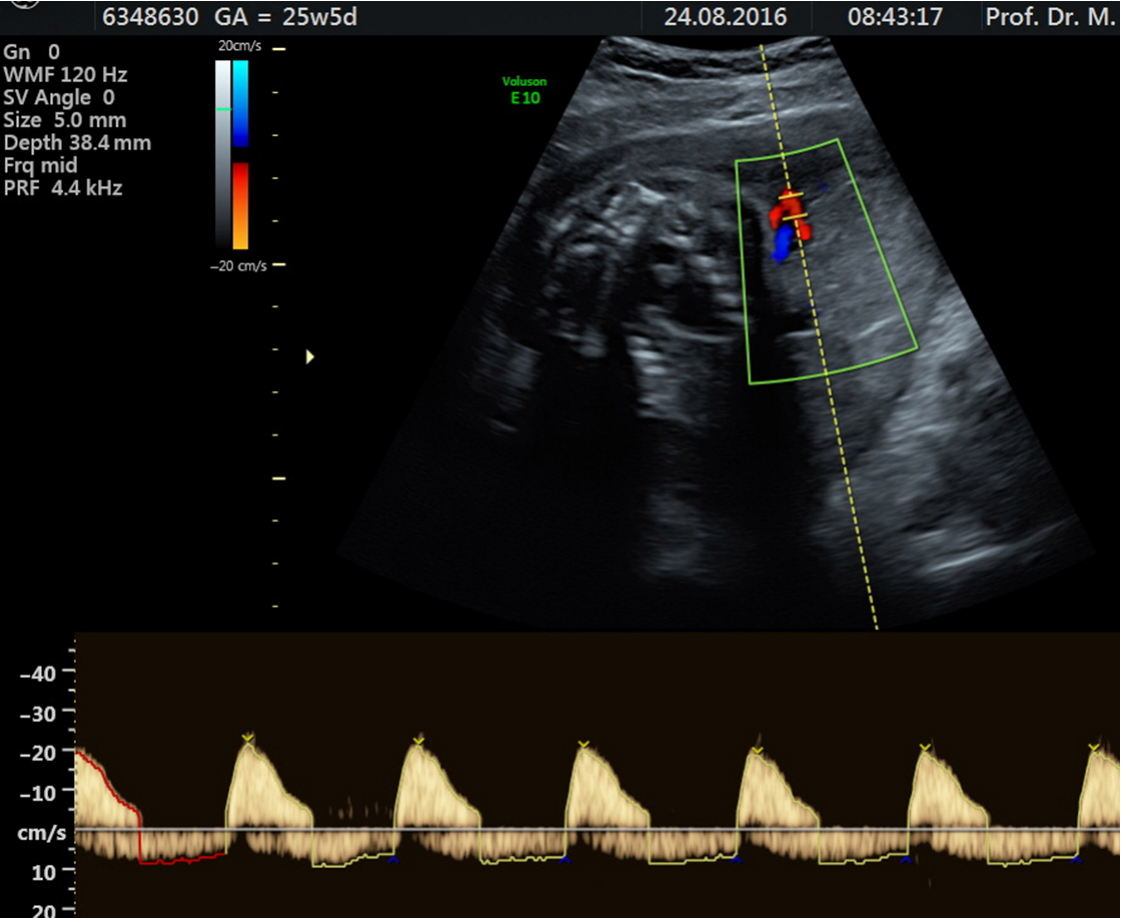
Doppler profiles of the umbilical artery and vein. Note the pulsation of the UV.
